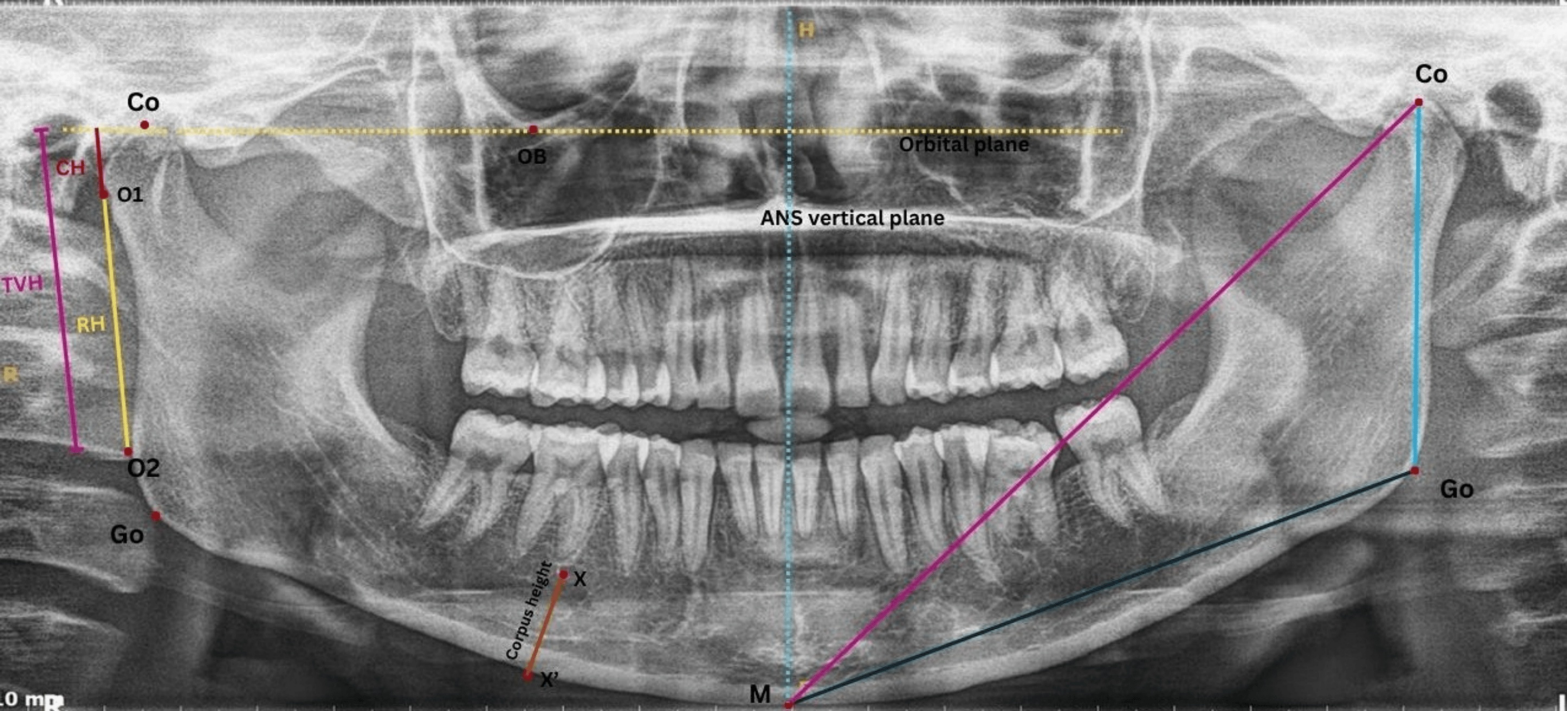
Linear parameters traced on an orthopantomogram CH represents condylar height (mm), measured as the red line from Line B through the Co point to O1. RH represents ramal height (mm), measured as the yellow line from O1 to O2 (Line A). TVH represents total vertical height (mm), measured as the purple line from Co to O2. Corpus height (mm) is measured as the red line from X (apex of the mesial root of the first molar) to X' (lower border of the mandible). Co-M represents total mandibular length (mm), measured as the purple line from Co to M. Go-M represents mandibular body length (mm), measured as the black line from Go to M. Co-Go represents combined condylar and ramal height (mm), measured as the blue line from Co to Go. Co: Condylion; M: midpoint on the inferior border of the mandible; Go: Gonion This figure represents the original orthopantomogram of the patient, obtained with the patient's permission, from the study.
